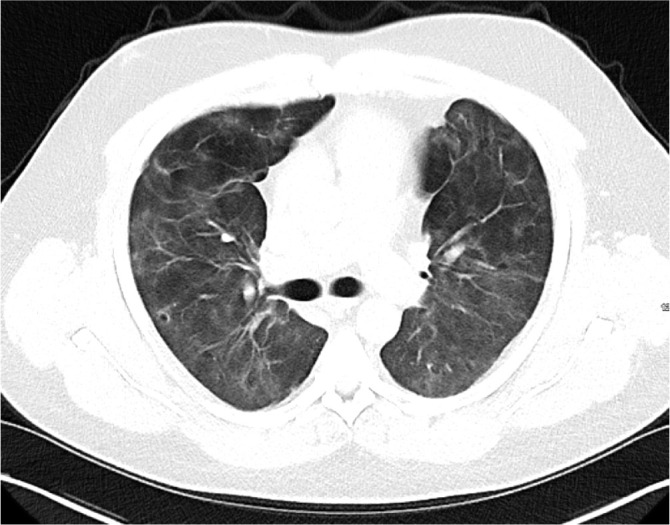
CT Thorax performed 2 years after initial presentation showing resolution of bilateral infiltrates. Ongoing upper lobe predominant reticulations and traction bronchiectasis were evident in keeping with interstitial fibrosis.
